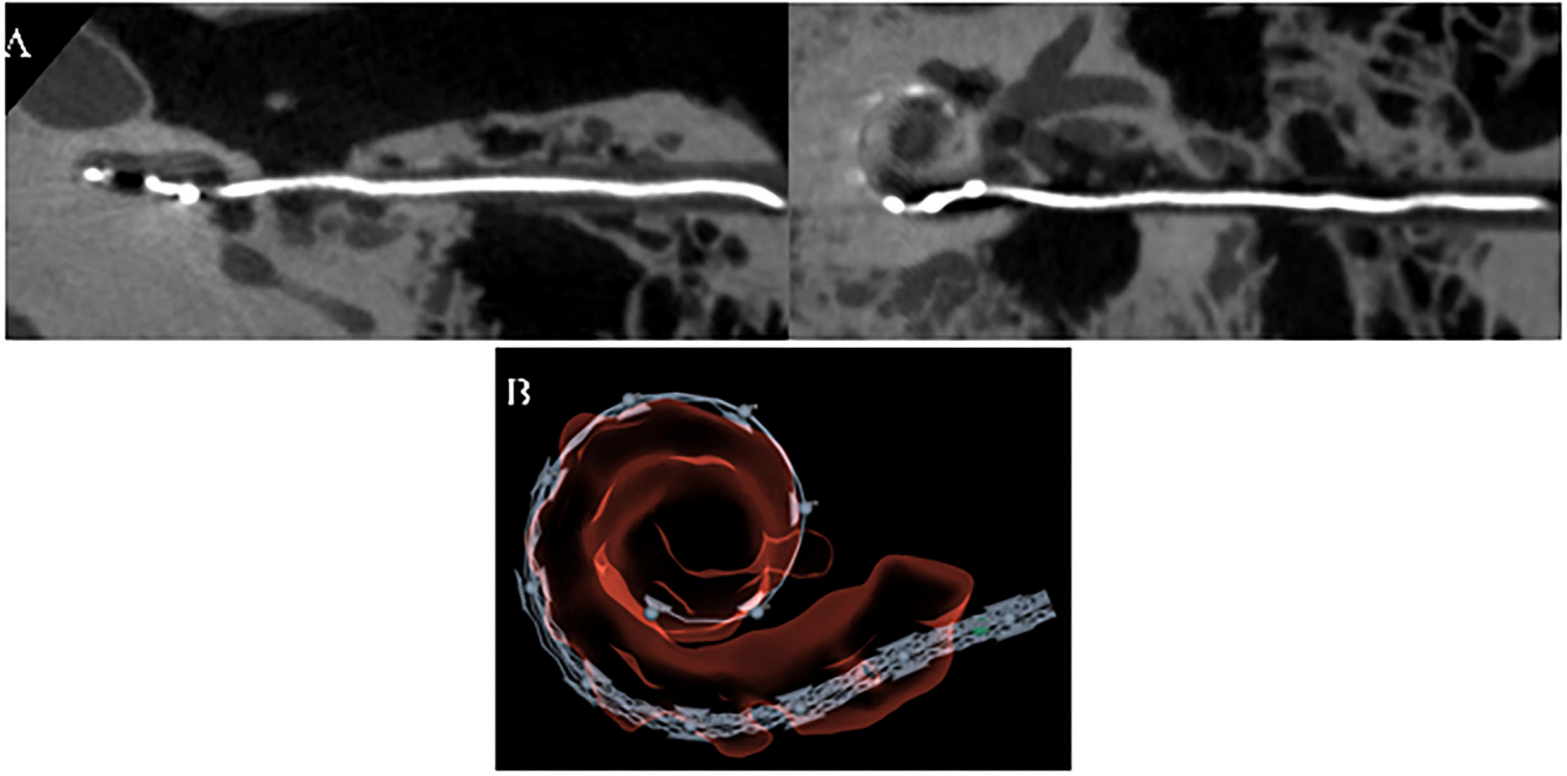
Post-op analysis of electrode insertion using (A). CBCT Siemens Artis Pheno and (B). OTOPLAN®.
